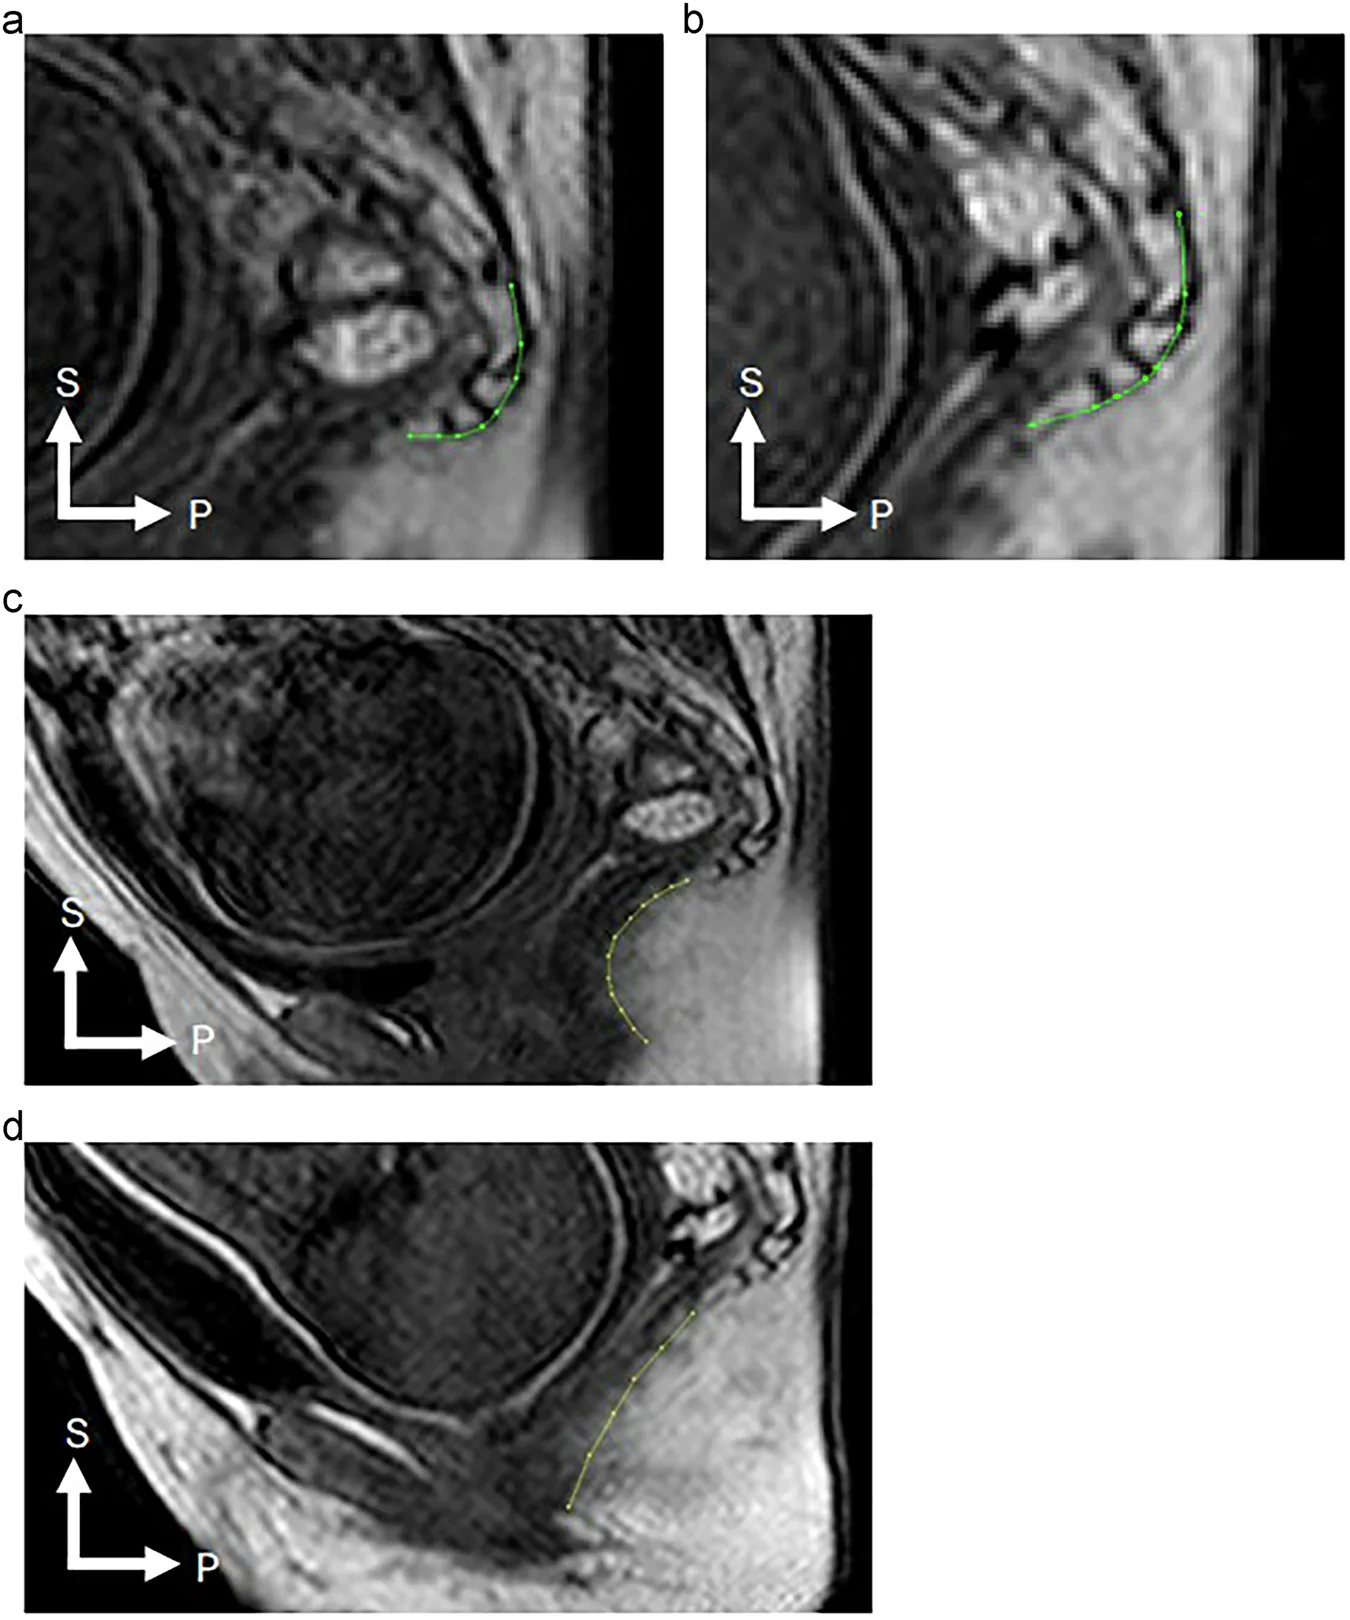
Sagittal MRI before the onset of labor (a, c) and during the SSL (b, d), patient #5 close-up of the coccyx before labor (a), which is concave anteriorly. During the SSL (b), the head is at the middle brim in the OA position, and the coccyx shows posterior nutation. The perineal raphe (in yellow) before the onset of labor is horizontal (c). During the SSL (d), the perineal raphe was pushed outward, resulting in a more linear appearance. MRI, Magnetic resonance imaging; OA, Occiput anterior; SSL, Second stage of labor
